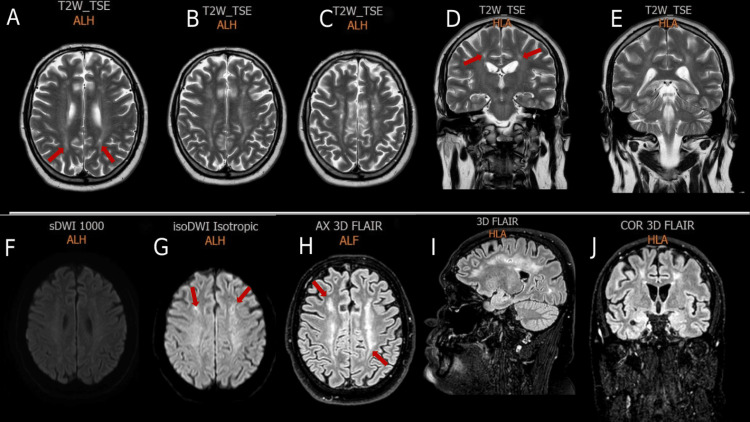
Third MRI of the brain showing a decrease in the size of the previous hyperintense lesions (red arrows) on T2W sequences (A, B, C, D, E), diffusion-weighted sequences (F, G), and FLAIR sequences (H, I, J). T2W = T2-weighted; FLAIR = fluid-attenuated inversion recovery
